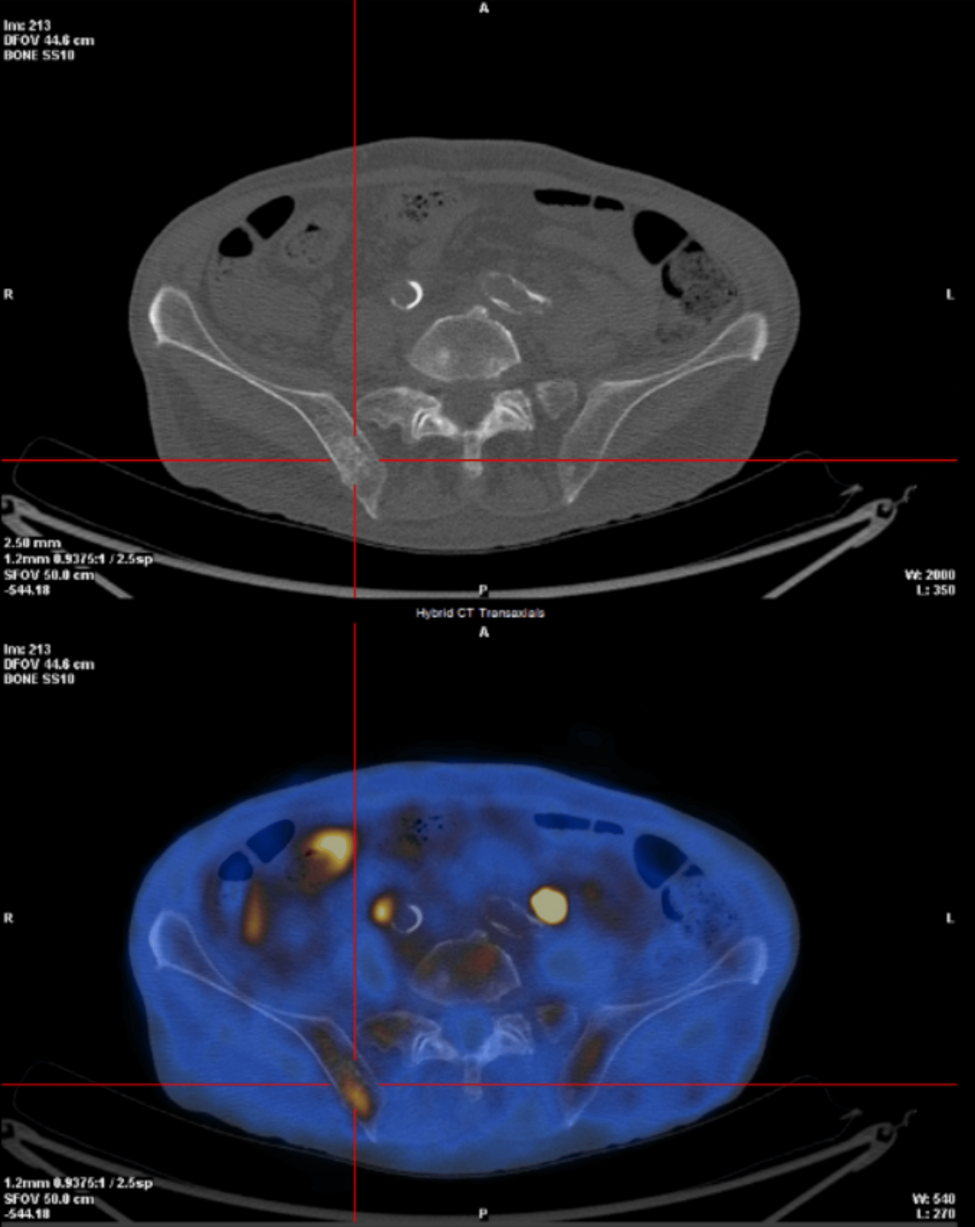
Whole body 18F-FDG PET-CT showing metabolic activity within the osteosclerosis in the right iliac bone FDG PET-CT: fluorodeoxyglucose positron emission tomography-computed tomography
